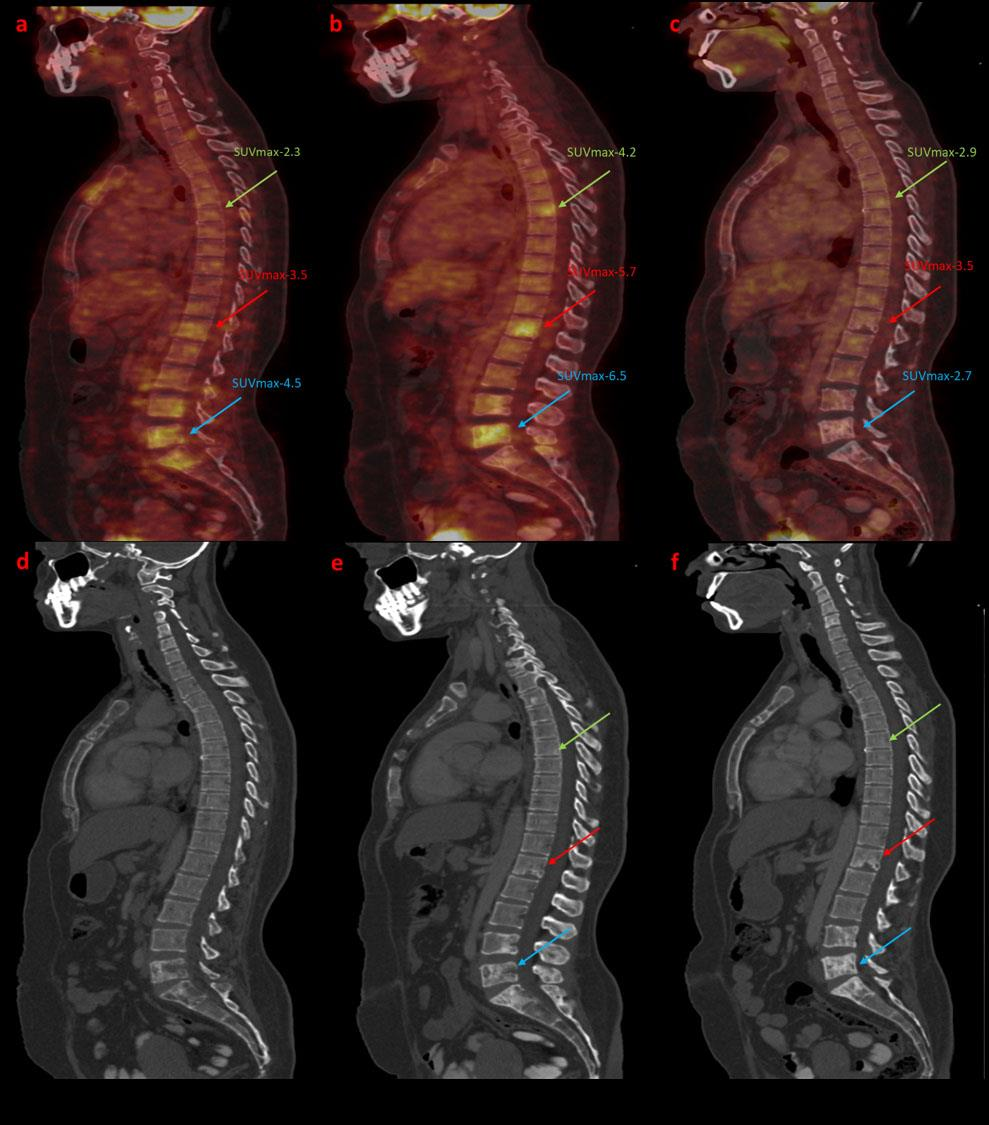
The initial FDG PET scan (Figure 1a-maximum intensity projection [MIP], 2a- sagittal fused PET-CT, 2d- sagittal CT) showed metabolically active mediastinal, right axillary, right supraclavicular, cervical, and retroperitoneal lymph nodes with extensive skeletal lesions. Interim FDG PET scan (Figure 1b-MIP, 2b-sagittal fused PET-CT) showed an increase in the FDG uptake and the SUV_max_ in the D7 (Green arrow), L1 (Red arrow), and L5 (Blue arrow) vertebrae. The SUV_max_ for vertebral lesions was 4.2, 5.7, and 6.5, respectively. Above mentioned corresponding vertebral lesions shows lytic -sclerotic changes on the CT (Figure 2e, Sagittal CT). The patient underwent an end of cycle FDG PET scan (Figure 1c-MIP, 2c-sagittal fused PET-CT) after four more cycles of chemotherapy. There was no significant FDG avidity in the previously noted lesion. Dense sclerotic changes were noted on CT (Figure 2f-sagittal CT)
